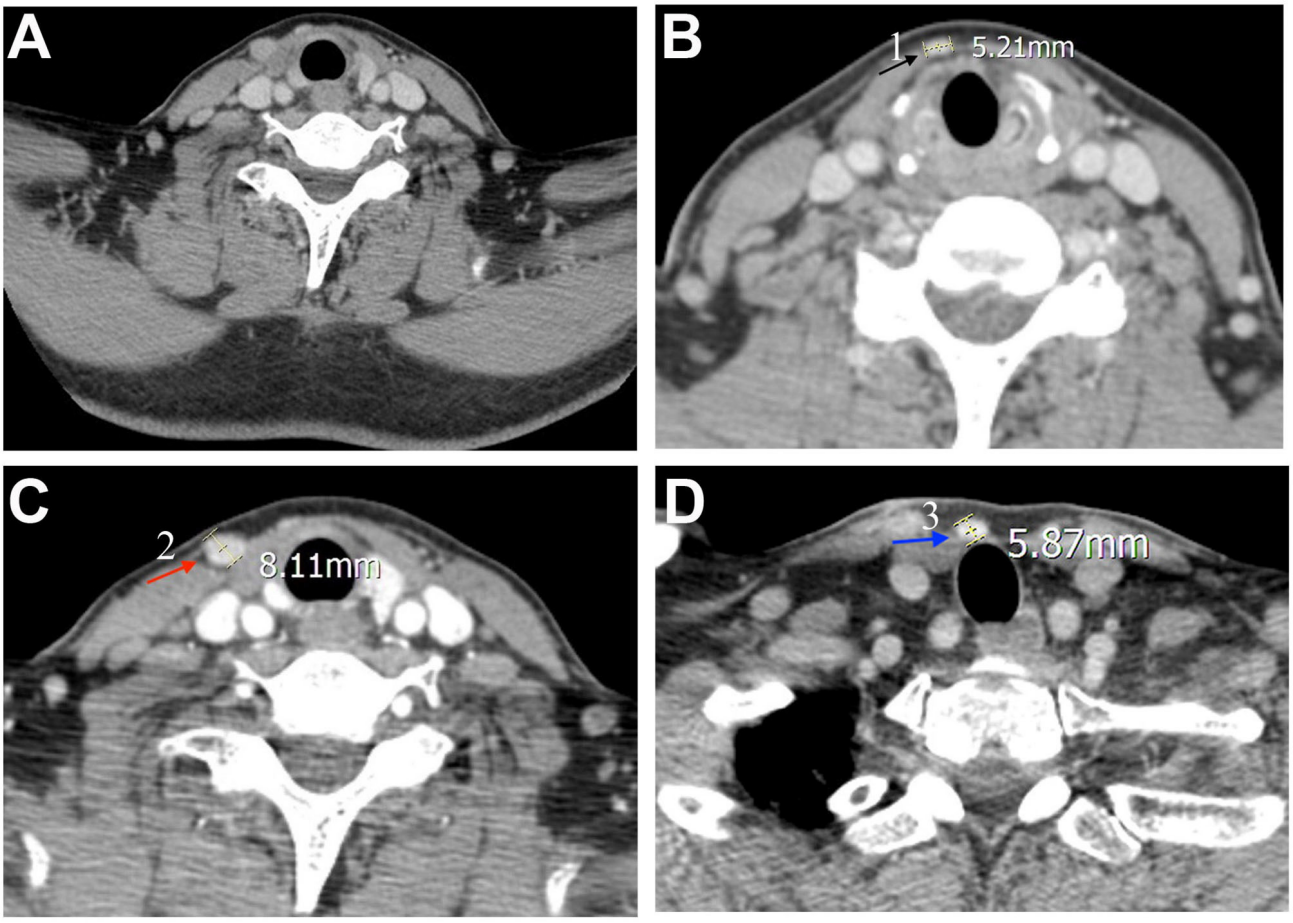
Pre-operative computed tomography findings. The neck enhanced computed tomography scans were observed (A) a general image of the neck, and (B–D) features of three nodules. The three nodules are annotated with black, red, and blue arrows, respectively, and corresponding nodules indicated by the arrow in Figure 1A.
